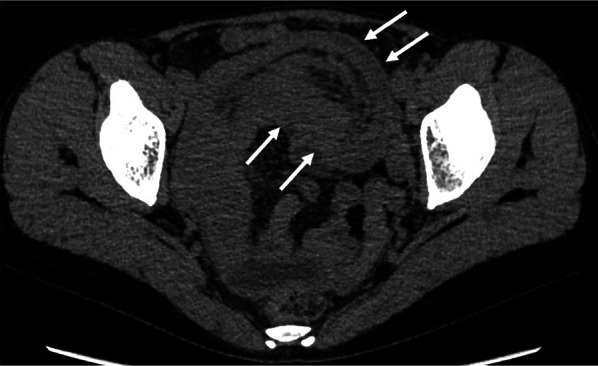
Computed tomography findings on the first admission. Computed tomography revealed intussusception (white arrows) in the jejunum
